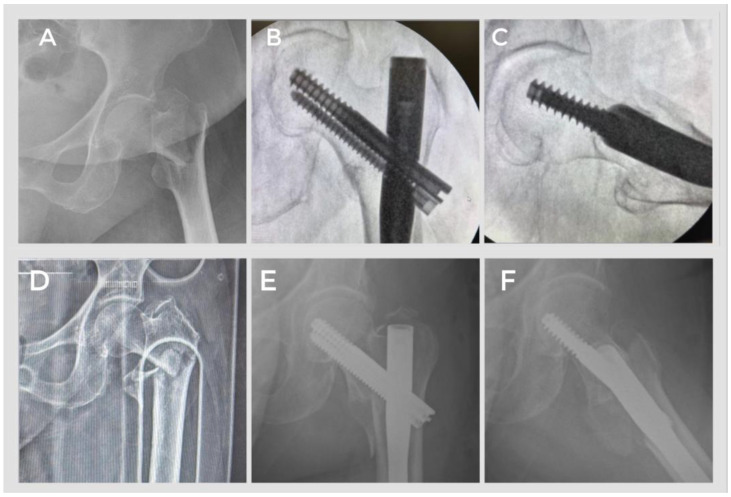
Preoperative and postoperative X-ray images of our patients. (A–C) A good reduction was achieved in our patient who was operated on for an intertrochanteric femur fracture of type AO 31A1.1. (D–F) Poor reduction was achieved in our patient who was operated on for an intertrochanteric femur fracture of type AO 31A3.3.
